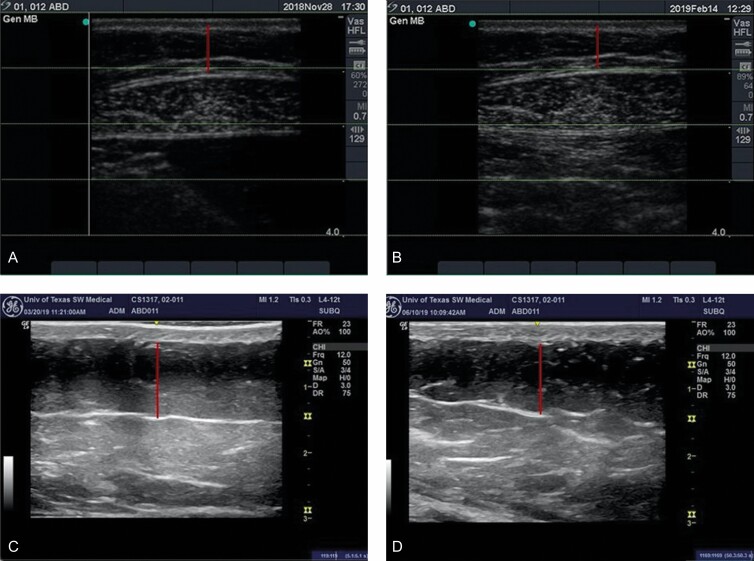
Ultrasound images showing a 12% (from 8.5 to 7.5 mm) decrease between baseline (A) At 12 weeks post-treatment (B) In adipose layer thickness at site 01 and a 9% (from 11.5 to 10.5 mm) decrease in adipose layer thickness between baseline (C) At 12 weeks post-treatment (D) in adipose layer thickness at site 02. Red lines indicate the adipose layer.
